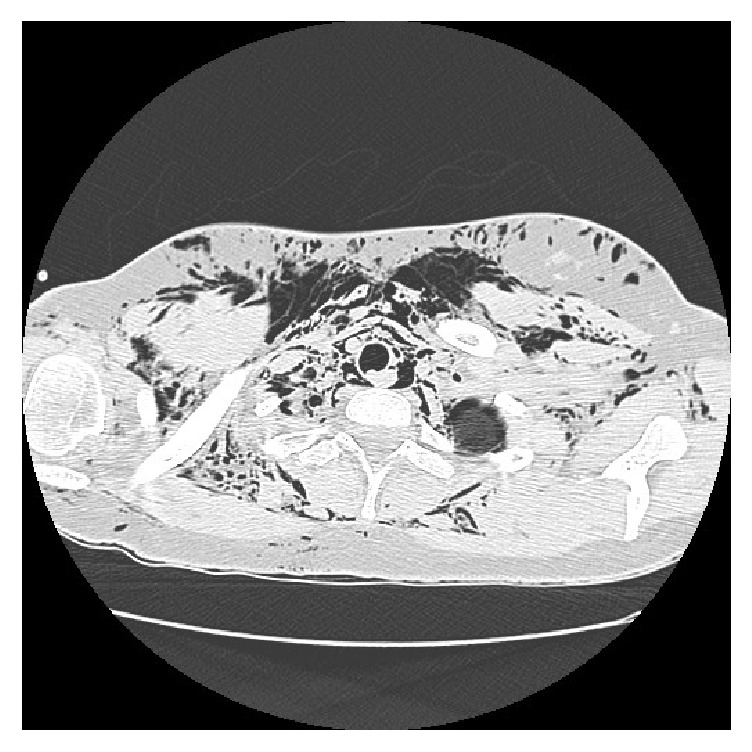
Cervical computed tomography showing extension of subcutaneous emphysema to the cervical region.
